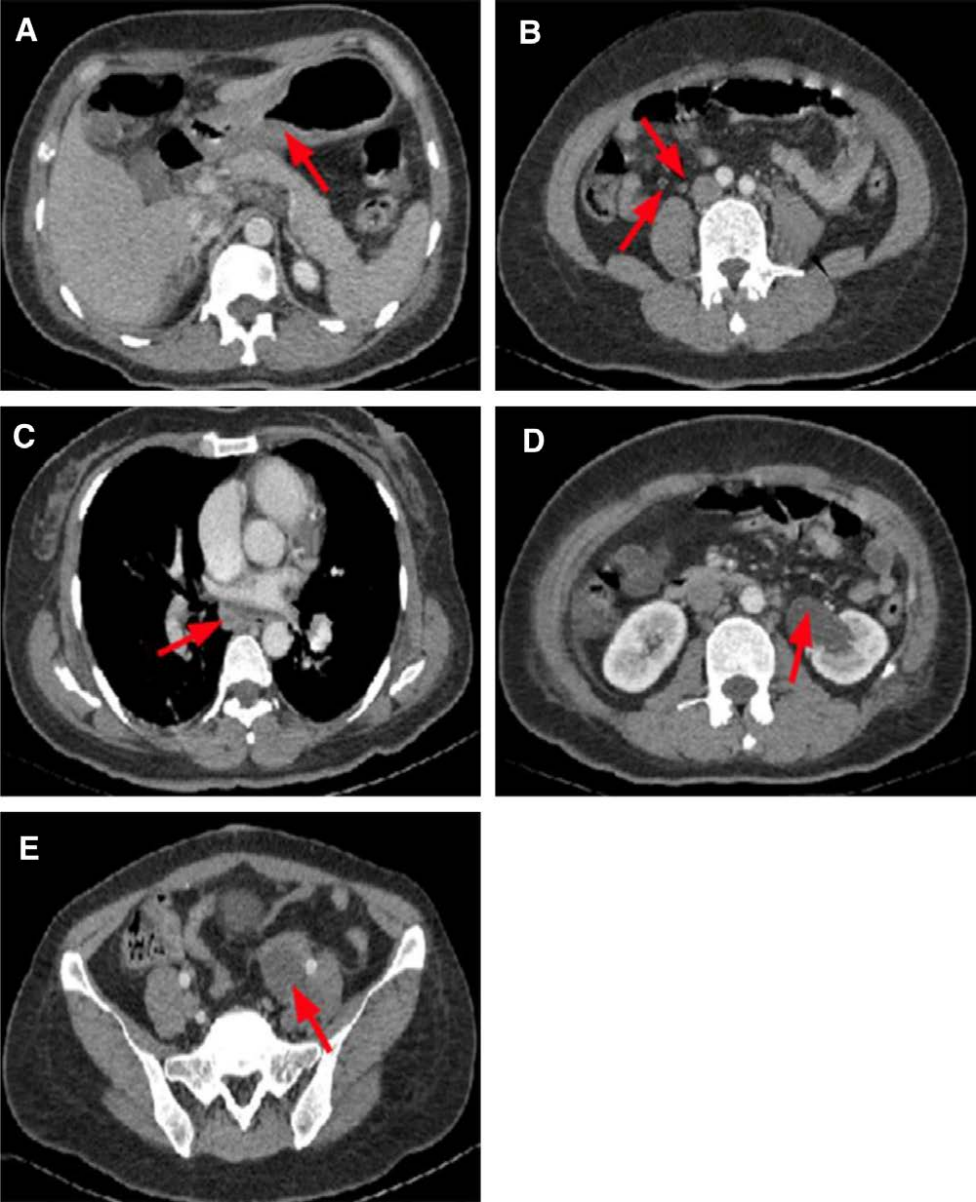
Abdominal enhanced CT. (A) Thickening of the stomach lining. (B) Retroperitoneal lymph node metastasis. (C) Mediastinal lymph node metastasis. (D) Renal pelvis dilatation. (E) Occupying lesion in the left adnexal region.
